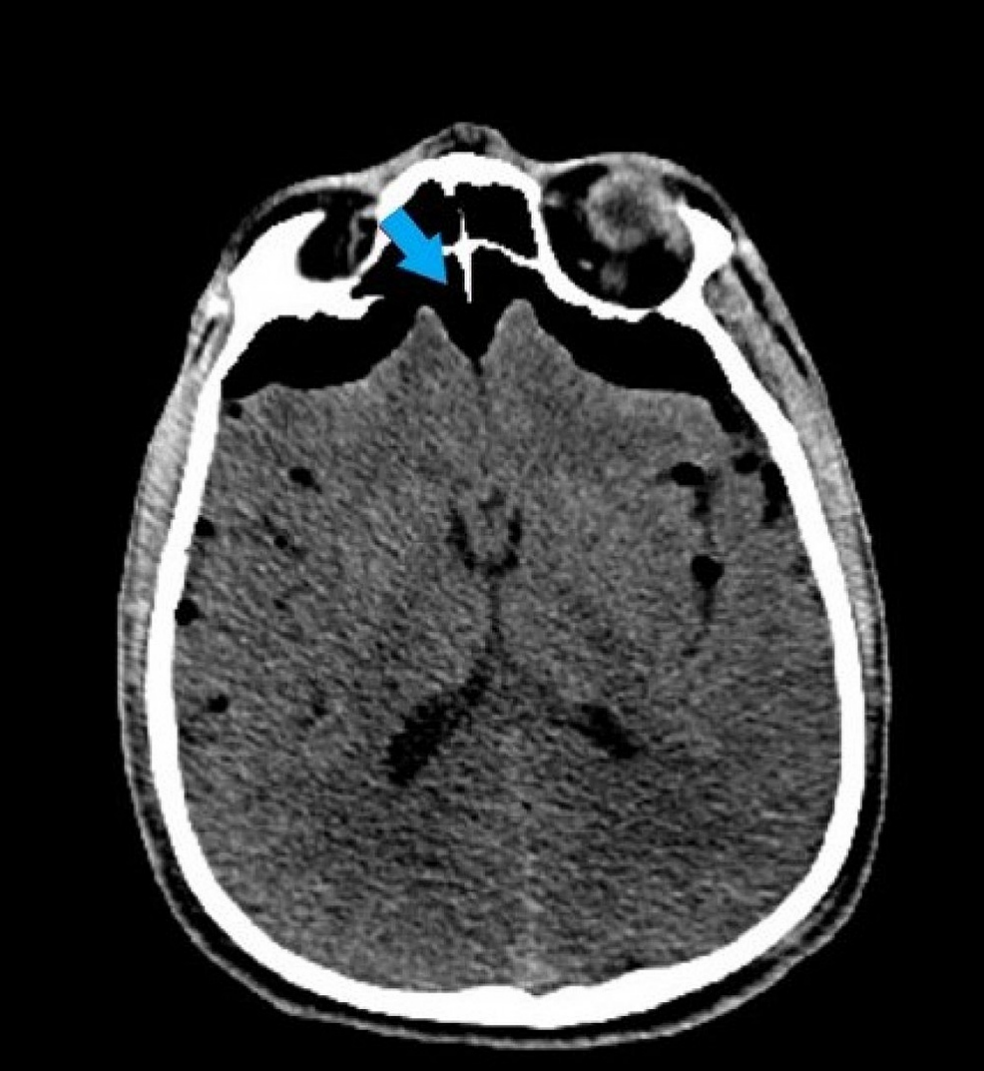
Noncontrast head CT through the frontal sinuses demonstrates the “Mount Fuji sign” of tension pneumocephalus (blue arrow). CT: computed tomography
